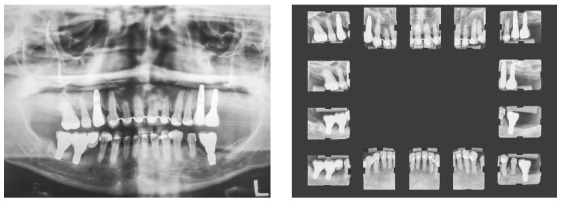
Retention radiographs of Case 3.
